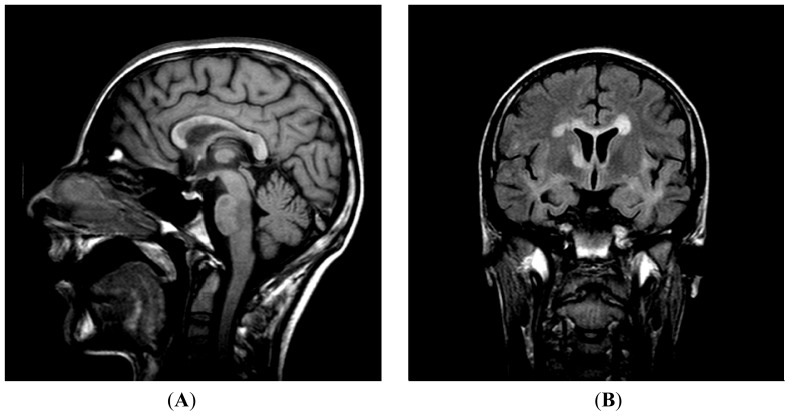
Brain MRI. (A) 2006 T1 Sagittal; (B) 2006 T2 Flair.
